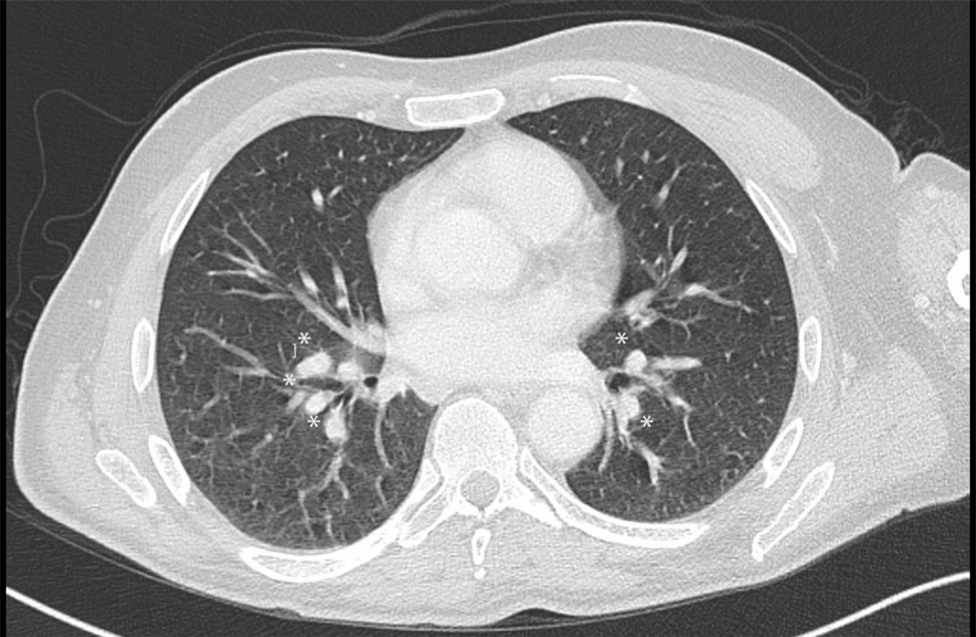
Axial chest CT scan showing bilateral hilar adenopathy (asterisks).
